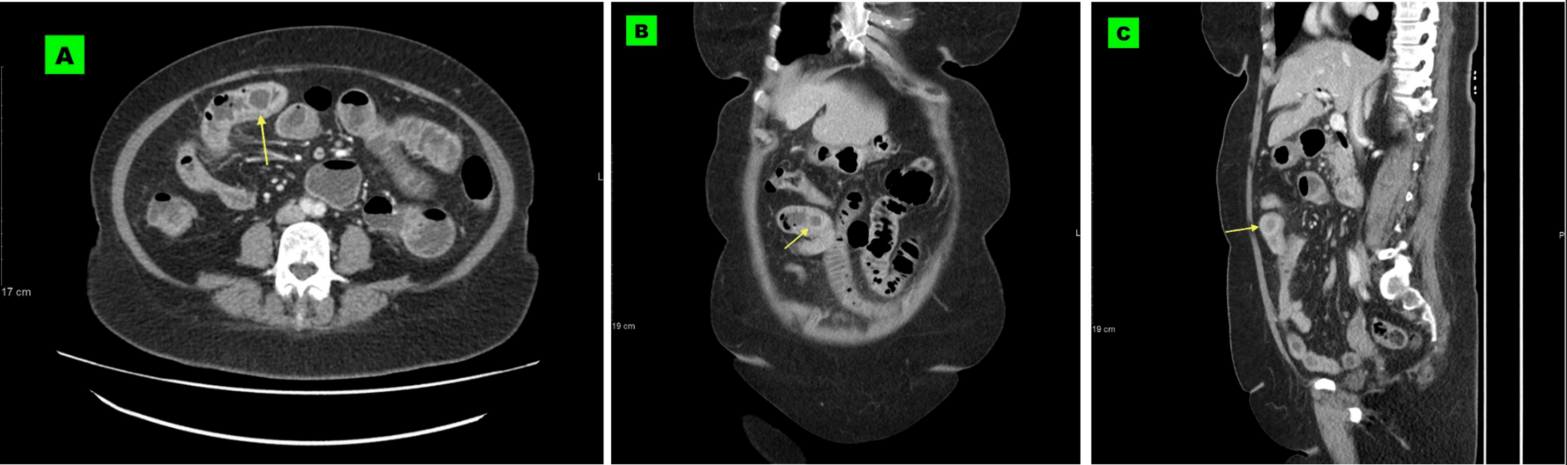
Axial (A), coronal (B), and sagittal (C) CT images show dilated small bowel loops with a well-defined isodense lesion at the transition point (arrows), which shows faint calcification best seen on the sagittal reconstruction. This was surgically proven to be a large gallstone CT: Computed tomography
